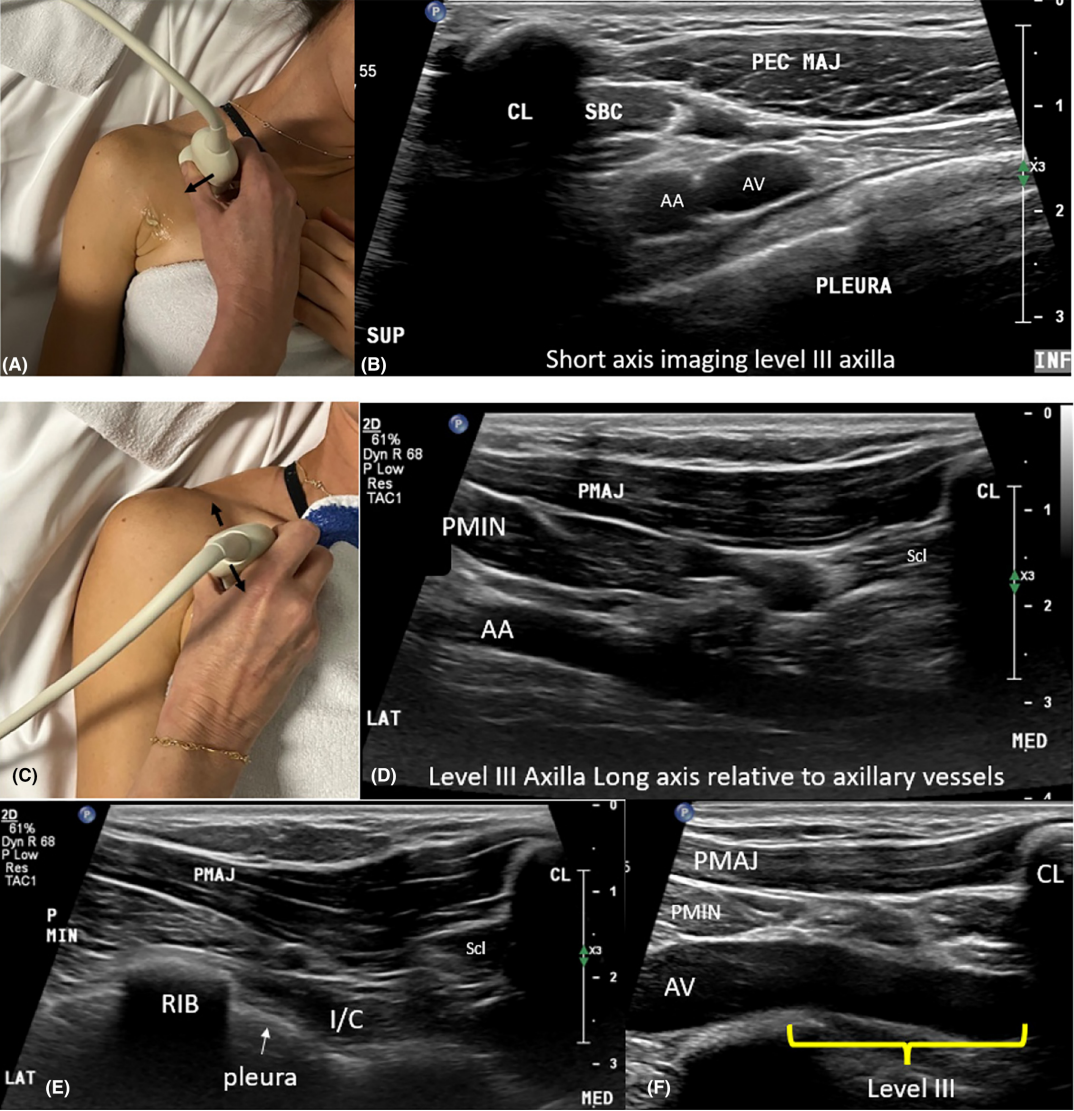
Sonographic imaging of level III of the axilla (Right axilla). (A). Transducer positioning and arm positioning for short‐axis imaging level III. (B). Short‐axis sonographic image of level III of the axilla (axillary vessels seen in cross section). (C). Transducer positioning for long‐axis imaging level III. (D). Long‐axis sonographic image of level III demonstrating the long axis of part 1 of the axillary artery which is located within level III of the axilla. (E). Long‐axis image level III inferior level to the axillary vessels, demonstrating rib, pleura and intercostal (I/C) muscles. (F). Long‐axis image of axillary level III demonstrating the long axis of the axillary vein and outlining the extent of level III of the axilla. AA, axillary artery; AV, axillary vein; CL, clavicle; INF, inferior aspect of the image; LAT, lateral aspect of image; MED, medial aspect of the image; PEC MAJ/PMAJ, pectoralis major muscle; PMIN, pectoralis minor muscle; SBC/Scl = subclavius muscle; SUP, superior aspect of the image.
